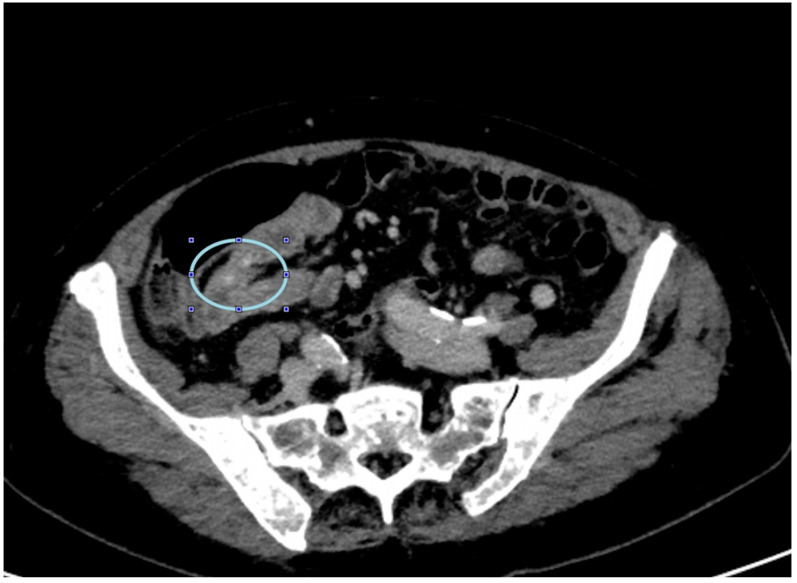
Angio CT contrast blushing in the proximal small bowel (first image).
